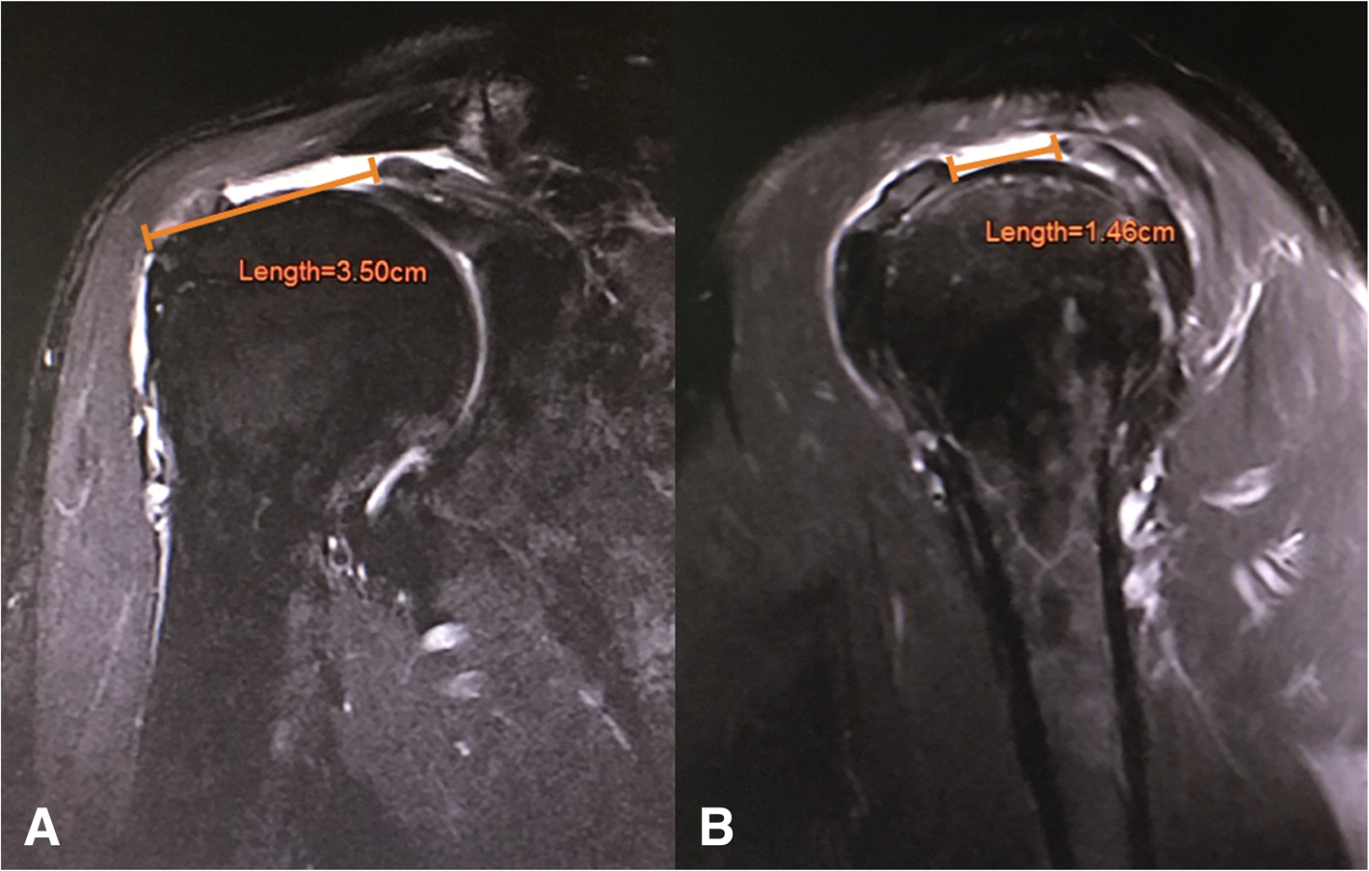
a From magnetic resonance imaging, mediolateral tear size was measured between the tendon edge and the lateral cortex of greater tuberosity in the coronal oblique cut, which the tendon most retracted medially. b Anteroposterior tear size was measured between the anterior tendon edge to the posterior tendon edge in the sagittal oblique cut, which showed the longest distance
